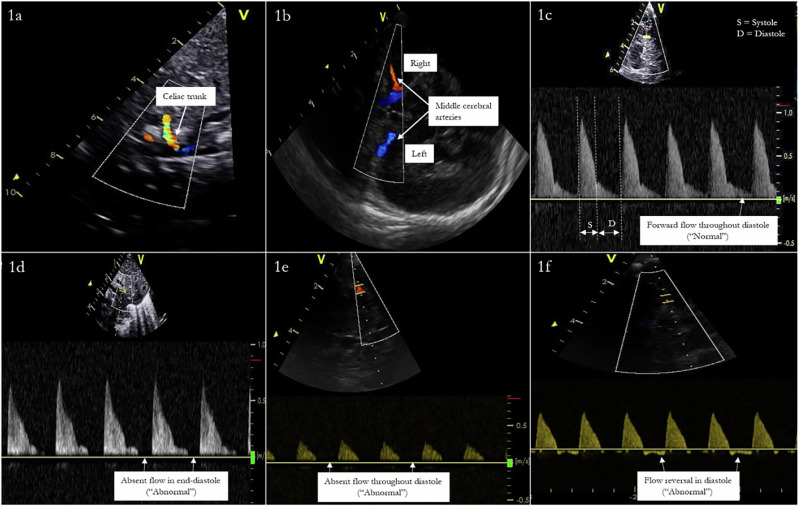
Doppler flow waveform of the celiac artery/trunk (CeT). Superior mesenteric artery (SMA), middle cerebral artery (MCA) and post-ductal descending aorta. Adapted from Keusters et al 2021 demonstrating the sagittal view of the celiac trunk (CeT) branching off the abdominal aorta (a), The Middle cerebral artery (MCA) (b). Forward flow in the MCA is depicted in (c) and abnormal flow (d) and absent end diastolic flow (e) and end diastolic flow reversal (f).
